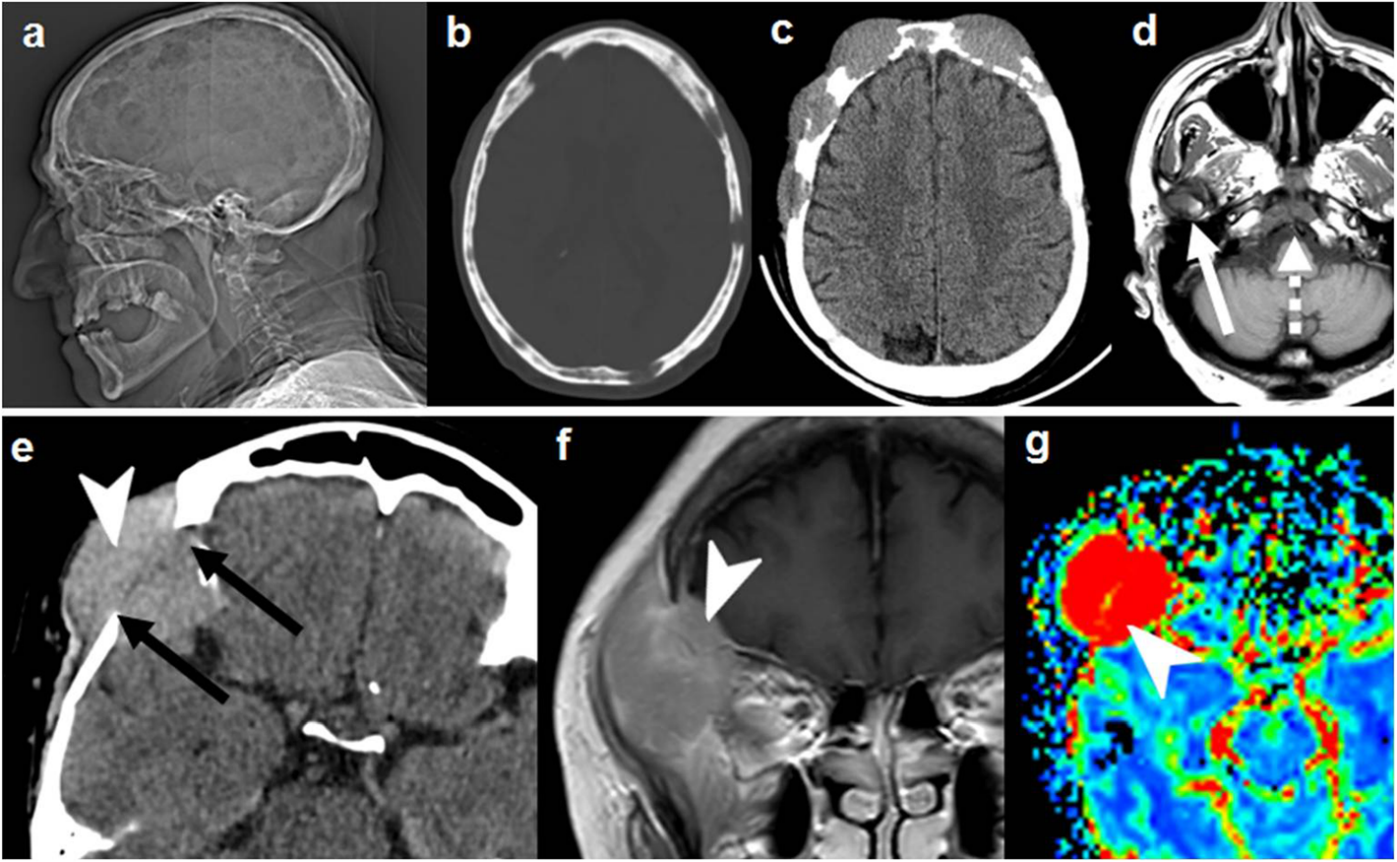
Myeloma and plasmacytoma. Upper row. Myeloma. X-ray (a) NECT (b, c) and T1WI (d). Multiple “punched-out” lytic lesions with variable soft tissue components (a–c). Mandibular lesion suggesting myeloma instead of metastases (arrow in d). Note also the clival lesion (dashed arrow in d). Lower row. Plasmacytoma. NECT (e), T1WI post-contrast (f), and DSC-PWI (g). Single purely lytic transdiploic lesion with narrow zone of transition (arrows in e), hyperdense, hyperenhancing, and hypervascular (arrowheads respectively in e–g)
